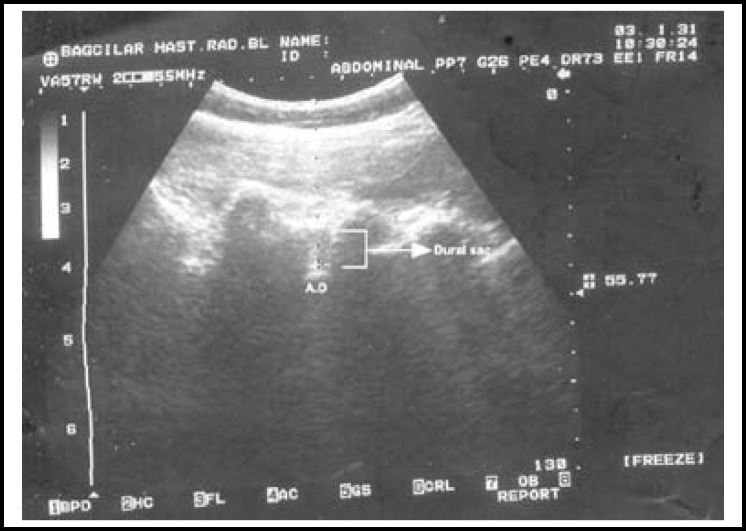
Ultrasonic image of sonoanatomic structures in intervertebral space and skin-dura mater distance.
